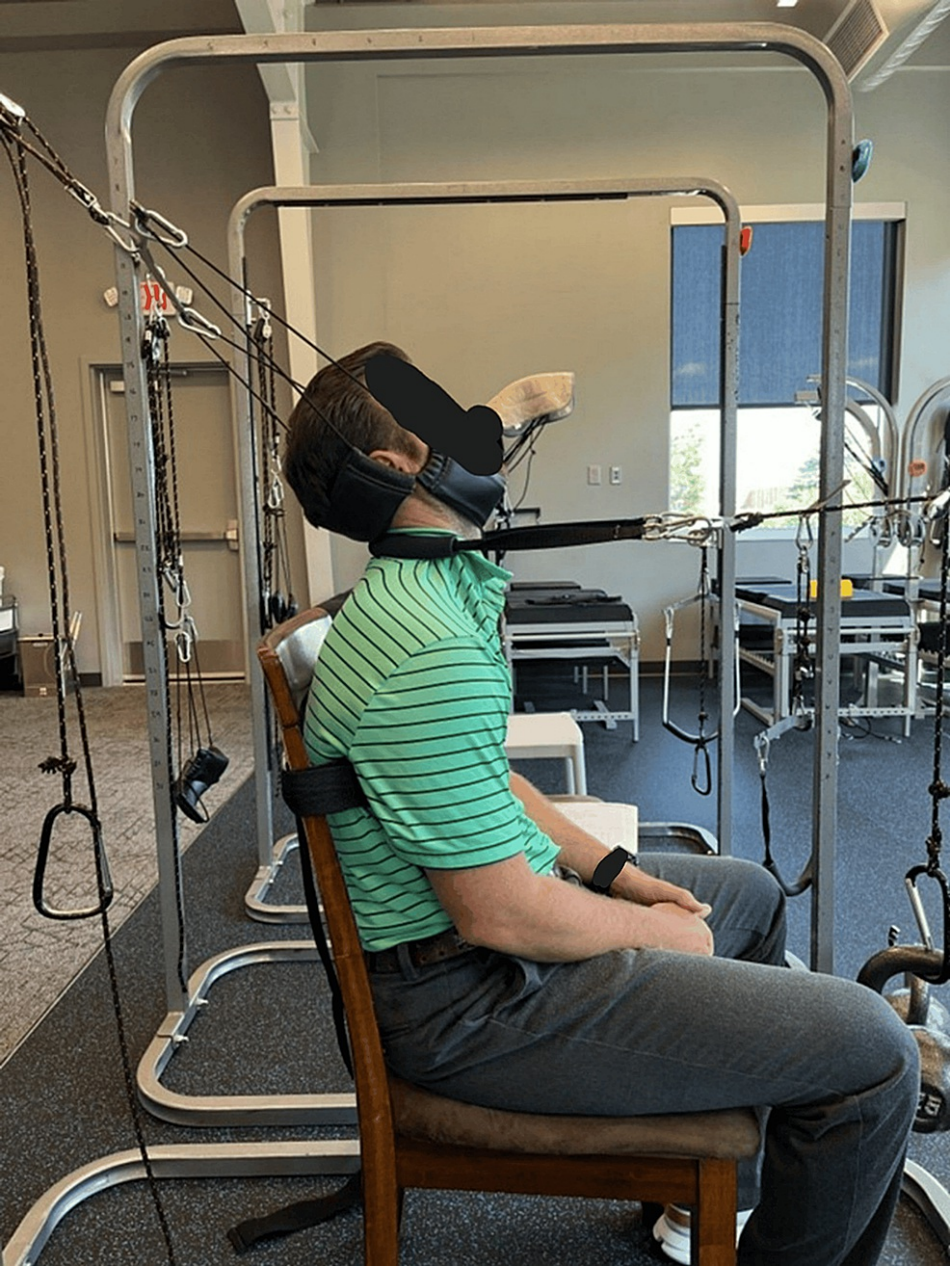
Two-way CET The patient is extended, and a slight distraction force posterior translates the head and extends the upper cervical spine, while a stronger force toward the front induces lordosis in the mid-cervical spine segments.  The chest is restricted from moving forward, and the patient exaggerates the thoracic spine to increase upper thoracic flexion and lower cervical extension. This traction is the mirror image of the radiographic findings CET: cervical extension traction
